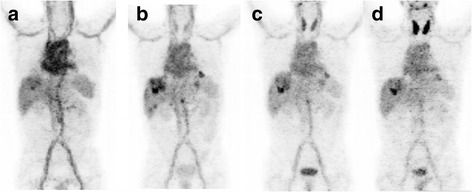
Patient 10 showed definite tumor accumulation in liver lesions at ~ 24 h and significant blood pool accumulation that decreased over time: 3 h (a), 22 h (b), 46 h (c), and 118 h (d). The highest SUVmax in the liver was 15.3 at 46 h. Normal organ distribution including the blood pool, liver, spleen, and kidney decreased over time. In contrast, free iodine accumulation in the thyroid increased over time
